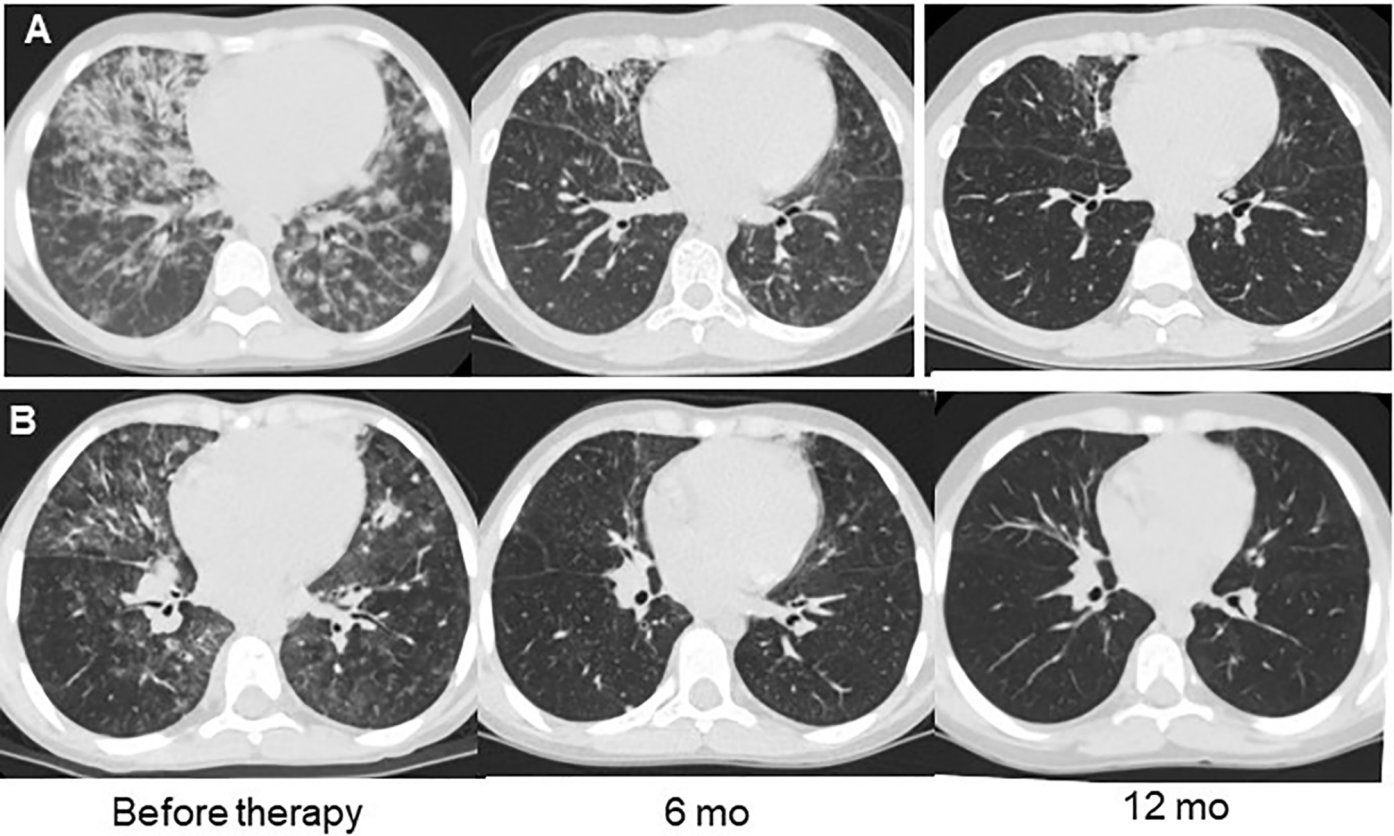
Examples of lung CT dynamics in the course of rituximab therapy. (A) – P8 with DiGeorge syndrome (del22); (B) – P14 with combined immunodeficiency with unknown genetic defect.
